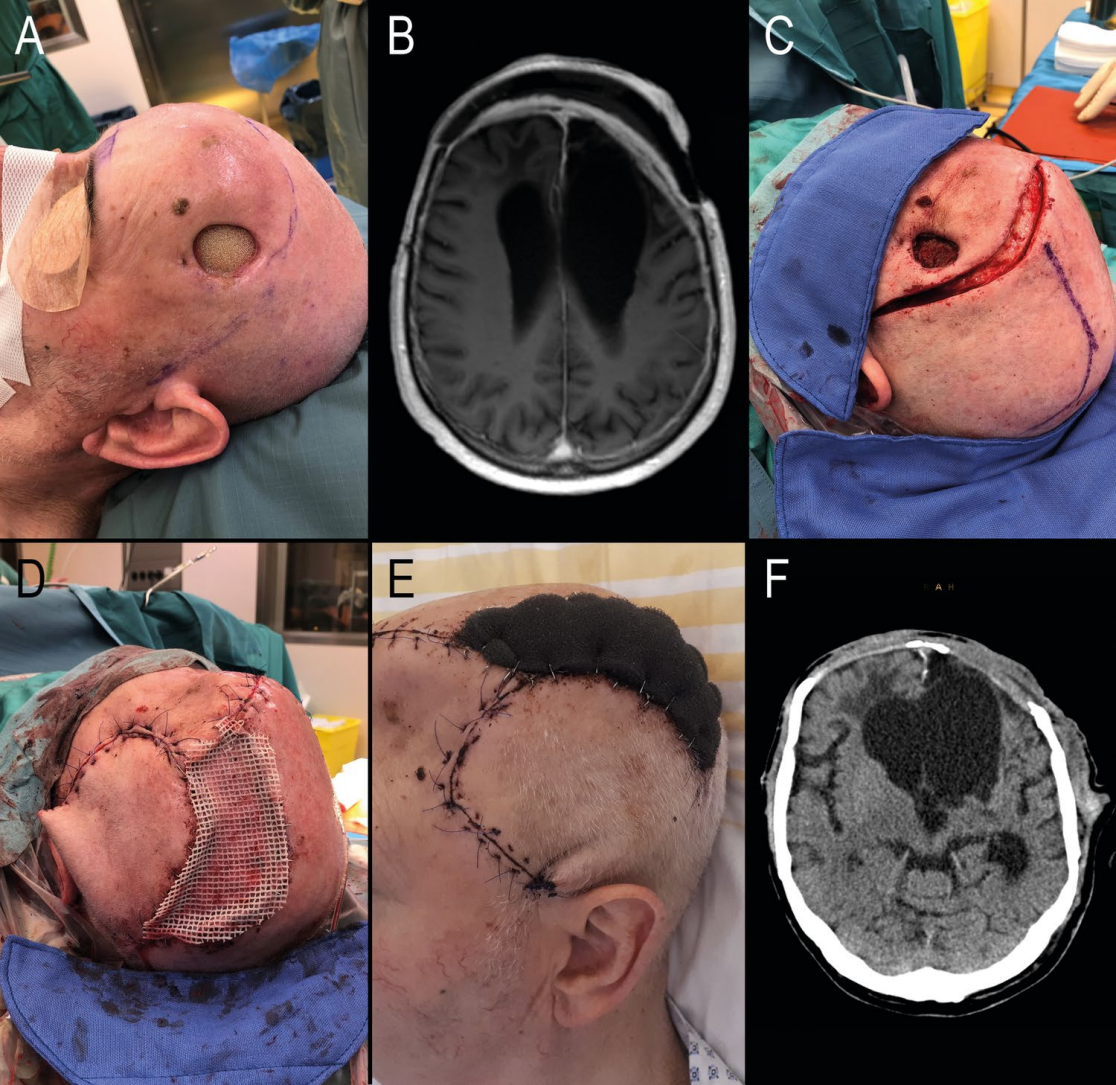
Patient 17: A 76-year-old male with a history of a large bifrontal solitary fibrous tumor (formerly known as hemangiopericytoma), classified as CNS WHO grade 2, treated with multiple surgeries for pneumocephalus and wound healing deficiencies, as well as tumor irradiation 10 years ago. The early postoperative course was uneventful. According to a follow-up telephone survey, the patient was alive 14 months after surgery but did not attend a scheduled outpatient appointment, making it impossible to evaluate his neurological status. A Preoperative view showing a left frontal gaping lesion of approximately 5 cm², exposing the underlying bone allograft. B Preoperative MRI scan revealing strong bifrontal epidural contrast enhancement, indicating infectious changes. C Intraoperative view after removal of the bone flap and before complete skin excision. D End-of-surgery view showing a left occipital rotation flap and split-thickness skin graft covered by fat gauze. E Early postoperative aspect with a well-vascularized skin flap and sunken skin over the bifrontal bone defect F Early postoperative CT scan
